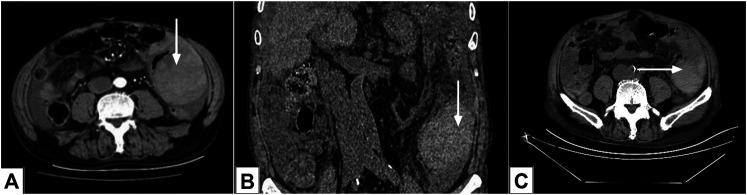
Abdominal computed tomography images. (A, B) Contrast-enhanced computed tomography (CT) images showing a hematoma of the colon (arrows) on March 23, 2018; C: CT image revealed that the colon hematoma had been absorbed (arrow) on March 27, 2018.
